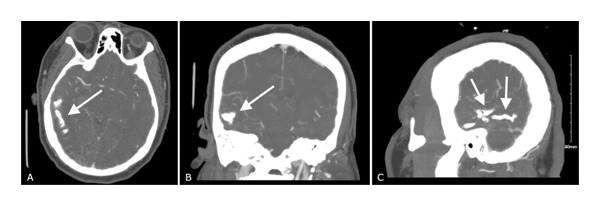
A CT venography of the head demonstrating findings characteristic of Sturge–Weber syndrome of leptomeningeal angiomatosis and gyriform calcifications involving the right posterior cerebral hemisphere as seen in the (A) axial, (B) coronal, and (C) sagittal views.
